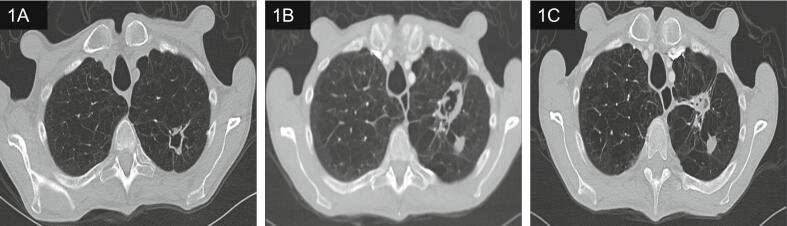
(a). CT chest shows an irregular cavitary lesion in the posterior left upper lobe measuring 3.18 mm × 18.7 mm with widespread small irregular noncalcified nodules in bilateral lung fields and moderate upper lobe predominant centrilobular emphysematous disease. (b). A new anterior left upper lobe cavitary lesion measuring 38.6 mm × 24.0 mm with the old posterior cavitary lesion with volume loss measuring 14 mm × 10.6 mm and the diffuse small noncalcified nodules. (c). Decrease in size of the anterior lesion measuring 28.7 mm × 14. 8 mm and stable posterior lesion measuring 13.5 mm × 11.9 mm.
